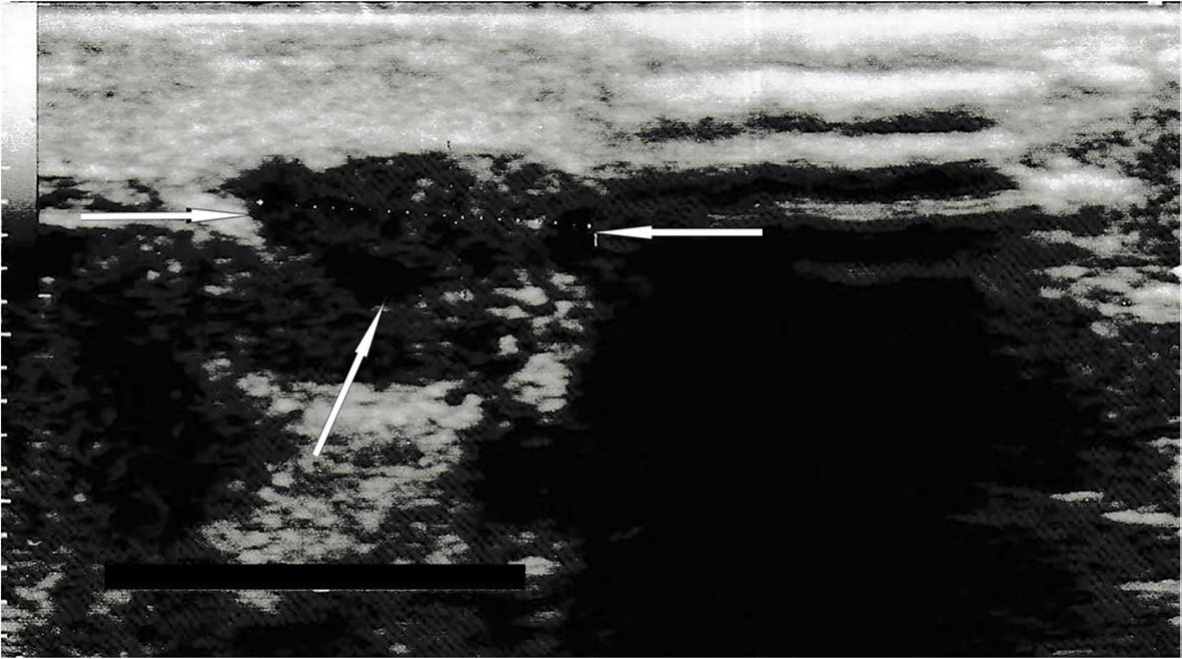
Ultrasound scan of the left side of the scrotum, showing small lesion with heterogeneous echogenicity
